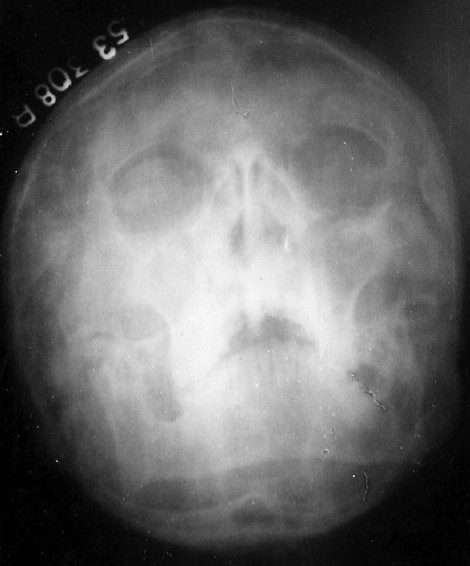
X-ray paranasal sinus showing maxillary sinusitis and bilateral hypoplastic frontal sinuses
